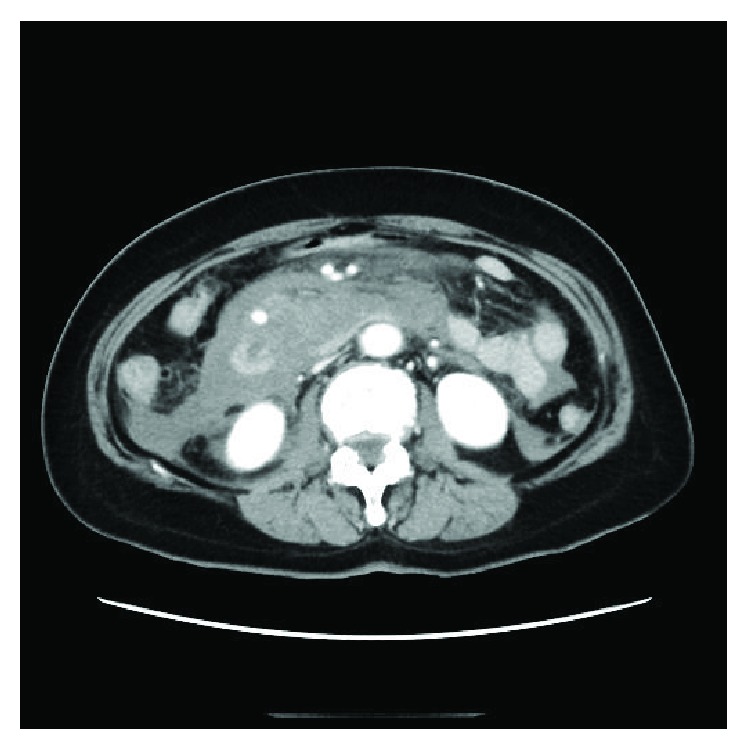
Case 1: a 66-year-old woman, CT scan showing a large amount of ascites and an aneurysm.
